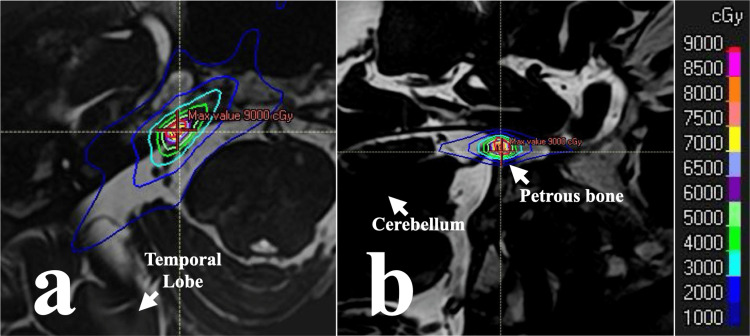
Dose distribution of SRS (Case 3). Isodose lines are shown relative to the maximum dose (100% = 90 Gy) on high-resolution T2WI. (a) Axial view and (b) sagittal view. SRS: stereotactic radiosurgery; T2WI: T2-weighted imaging.
